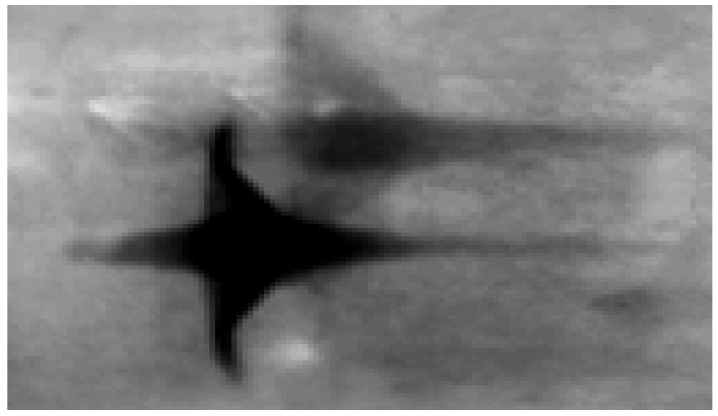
X-ray results of the high-density reactive jet formation.
